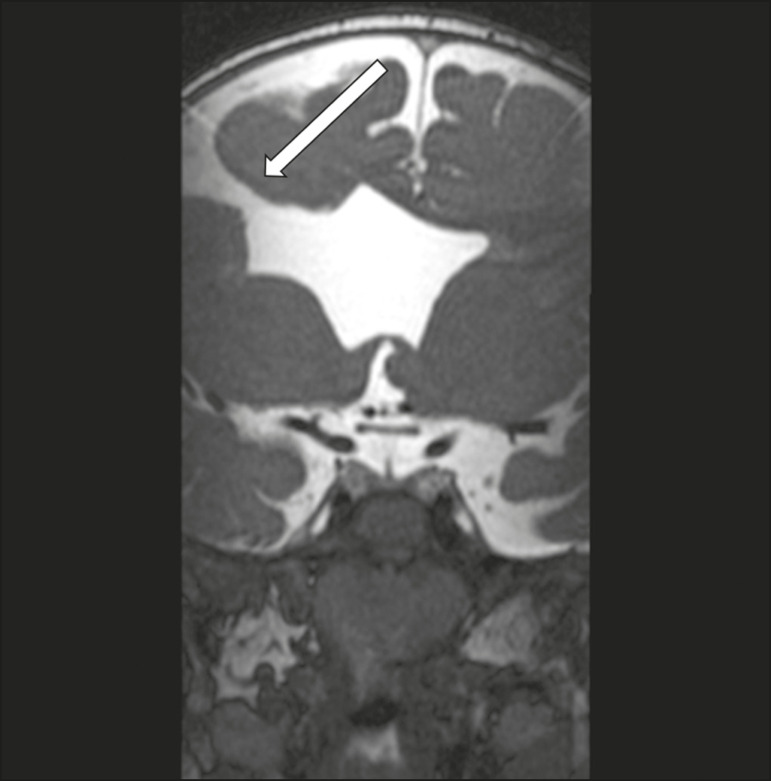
Septo-optic dysplasia plus. A 6-year-old male with eyelid myoclonus. Coronal T2-weighted MRI scan showing absence of the septum pellucidum and a wide cerebrospinal fluid-filled cleft (arrow) in the right frontal lobe (extending from the polar region to the medium convexity) and the anterior horn of the right lateral ventricle, wrapped in a thickened cortex. The right Sylvian fissure was flat and vertical, with thickened cortical segments, and there was, on the ipsilateral side, polymicrogyria of the insula and frontal operculum (not shown).
